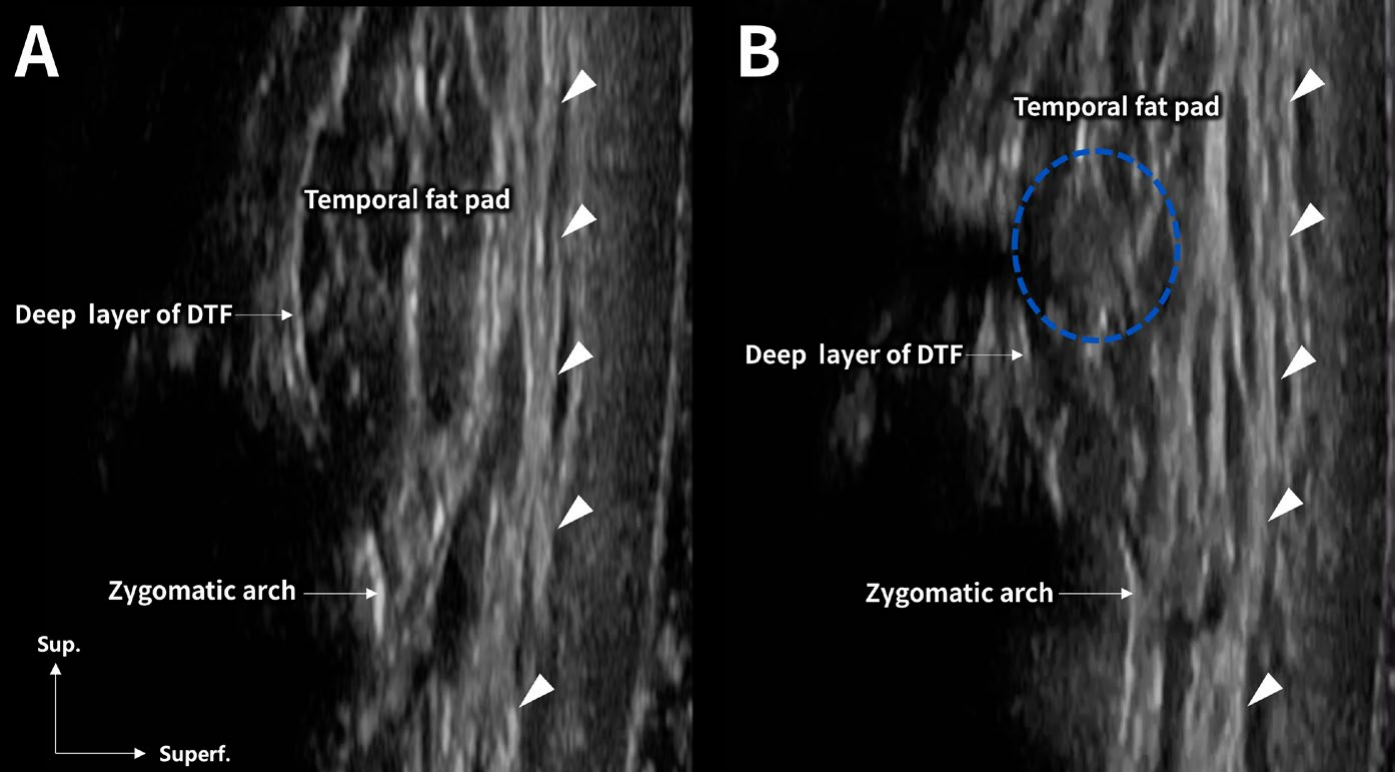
Before injection (A): Ultrasound image showing the superficial layer of the deep temporal fascia (DTF) prior to filler injection. After injection (B): Ultrasound image of the superficial layer of the DTF, demonstrating an upward shift and enhanced tension following filler injection, with the blue circular marker indicating the placement of poly‐D,L‐lactic acid hybrid hyaluronic acid filler (Juvelook Volume). The white arrowhead points to the superficial layer of the DTF.
